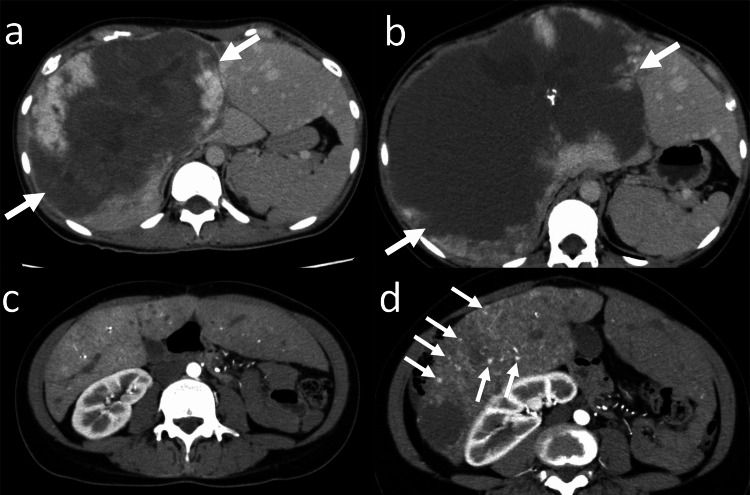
Enlargement of a hepatic hemangioma and hemangiomatosis in a 31-year-old woman (Case 1 in Table 1) (a) Axial delayed-phase computed tomography (CT) (baseline study) of the upper abdomen shows a hepatic hemangioma (arrows) with a diameter of 182 mm located in the right lobe and the medial segment of the liver. (b) Axial delayed-phase CT (final study) of the upper abdomen obtained at the 91-month follow-up shows enlargement of the hepatic hemangioma (arrows). The diameter of the hepatic hemangioma is 240 mm. (c) Axial arterial-phase CT (baseline study) at the right kidney shows no hepatic hemangiomatosis. (d) Axial arterial-phase CT (final study) at the right kidney shows multiple small foci of nodular enhancement (arrows) in the right lobe of the liver, consistent with hepatic hemangiomatosis.
